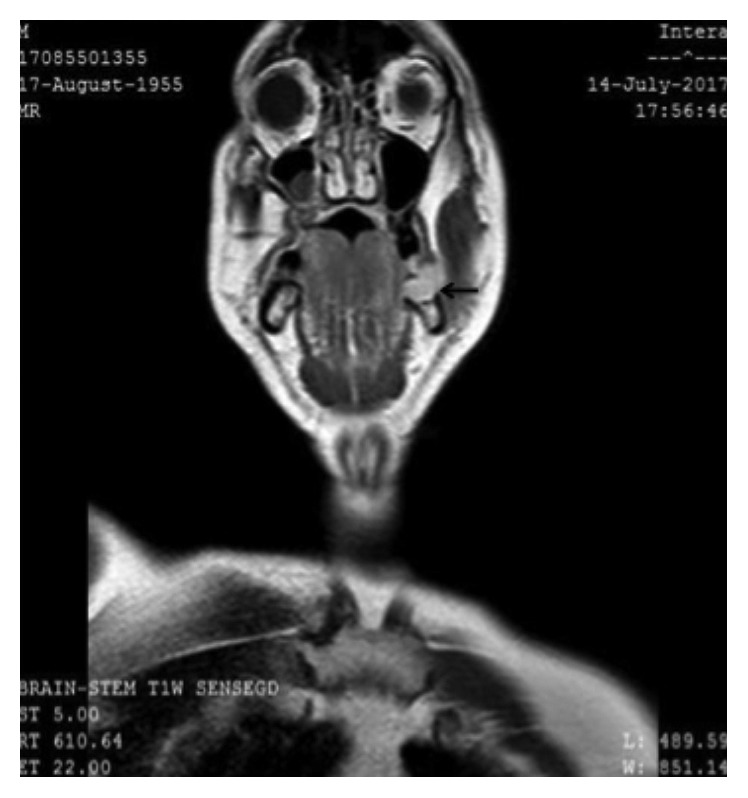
Imaging of the lesion in the internal surface of the mandible on the left in cranial MRI T1 with gadolinium (black arrow).
